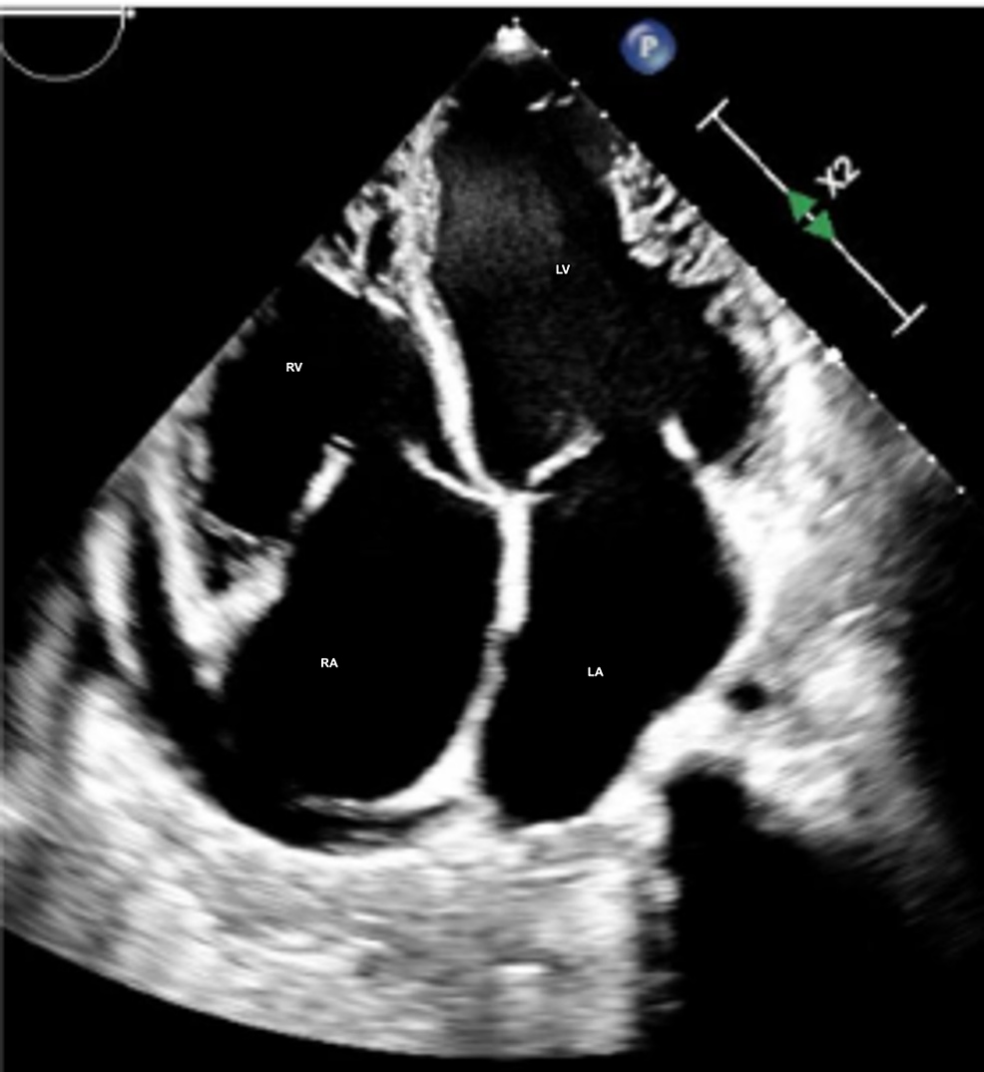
Left Ventricle (four-chamber view on echocardiogram) LV: left ventricle; RV: right ventricle; LA: left atrium; RA: right atrium
